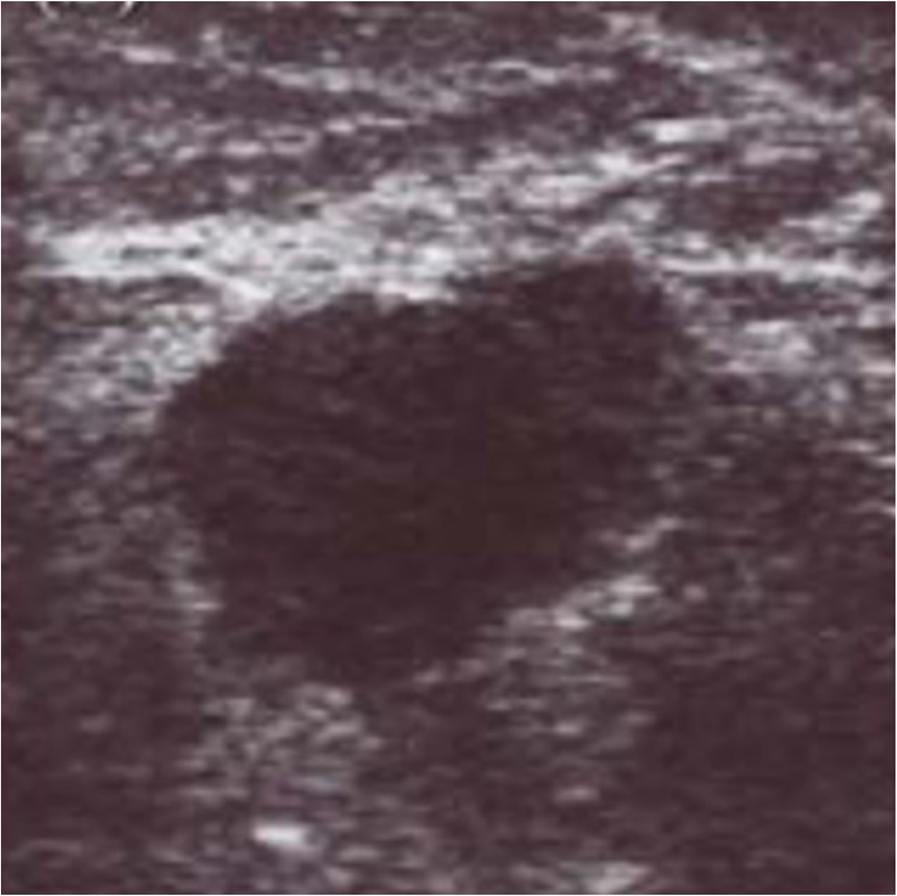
Ultrasound image of the tumor
